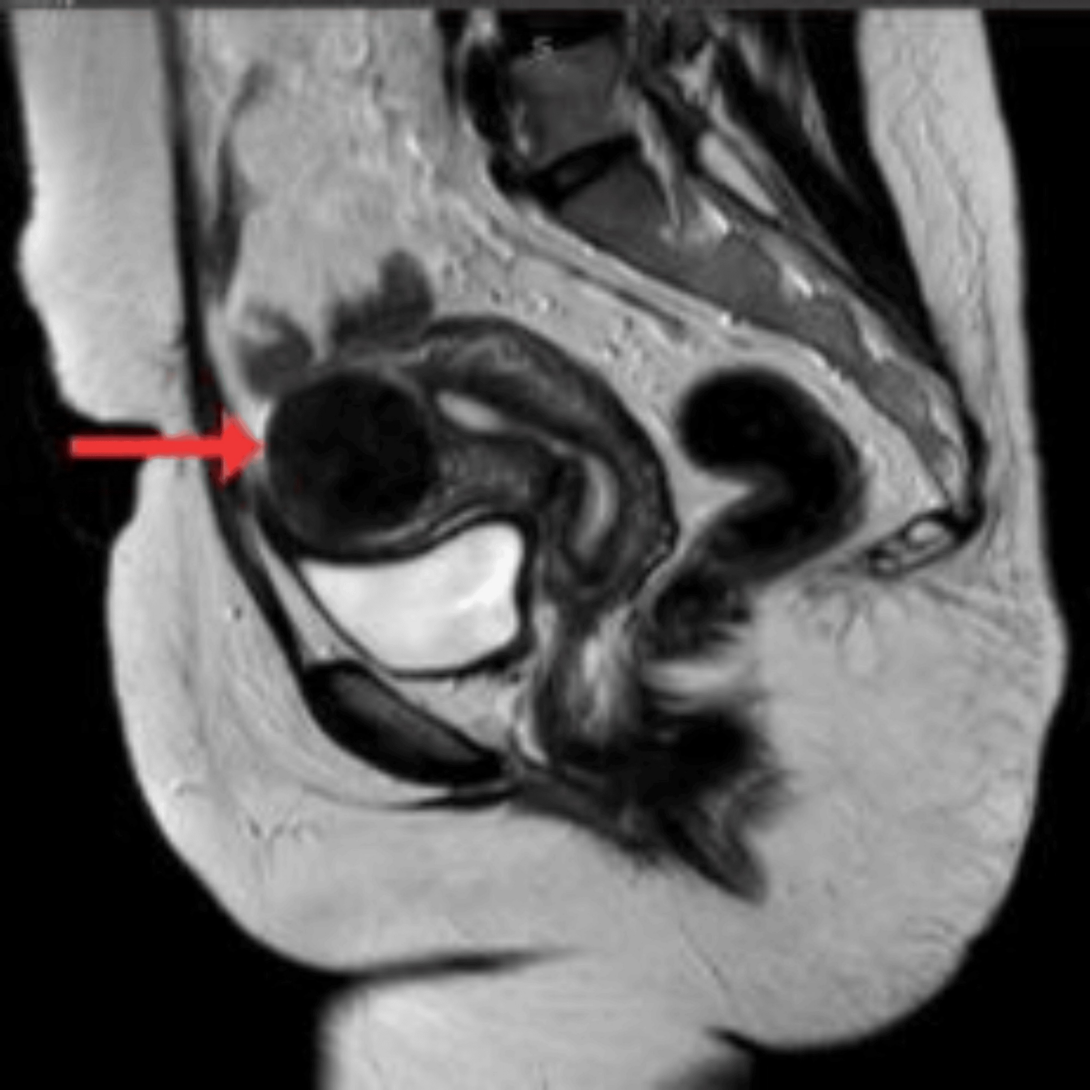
Sagittal T2-weighted MRI image of the pelvis showing a large, well-circumscribed heterogeneous mass (red arrow) with both cystic and solid components, arising from the left adnexal region. The mass demonstrates a hyperintense signal on T2, suggestive of fluid content, and displaces adjacent pelvic structures without clear evidence of infiltration, supporting a diagnosis of a potentially malignant ovarian lesion.
